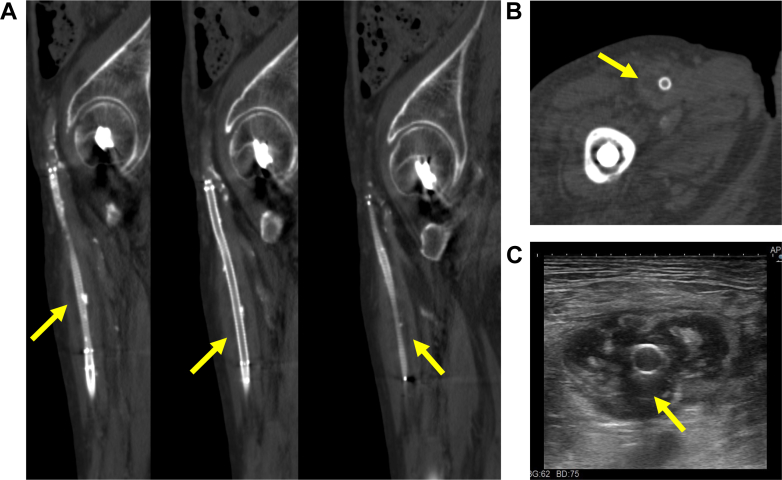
Ultrasound and Computed Tomography Findings CT scans and ultrasound reveal a low-density area around the stent graft (SG) (yellow arrows), suggesting possible abscess formation. (A) Sagittal and (B) cross-sectional CT scans demonstrate a low-density area around the SG. (C) A cross-sectional ultrasound shows an irregular low-echogenic structure surrounding the SG.
